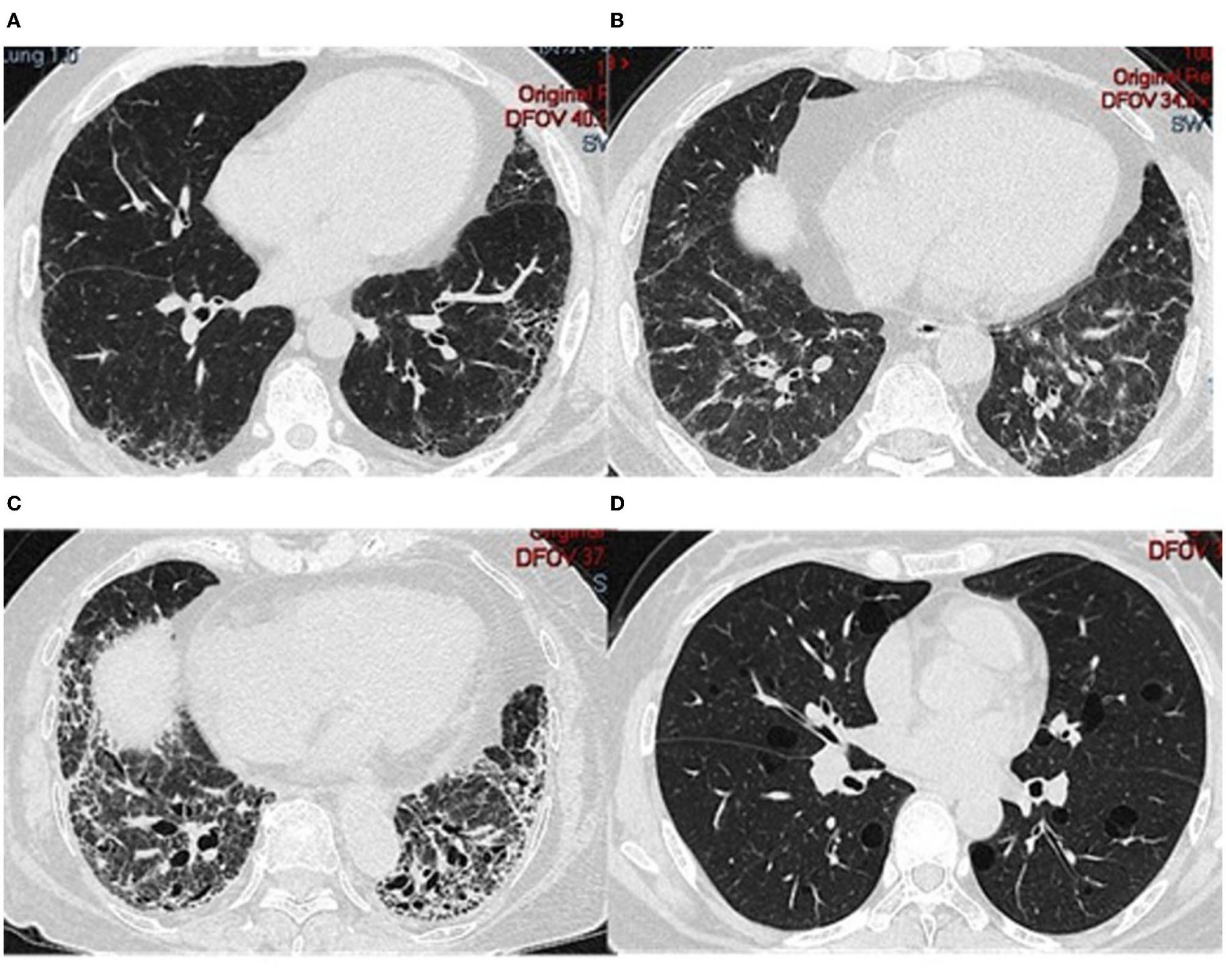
HRCT from different patients who tested positive for anti-PM/Scl. (A) 41-year-old man; (B) 52-year-old man; (C) 83-year-old woman; (D) 41-year-old woman.
